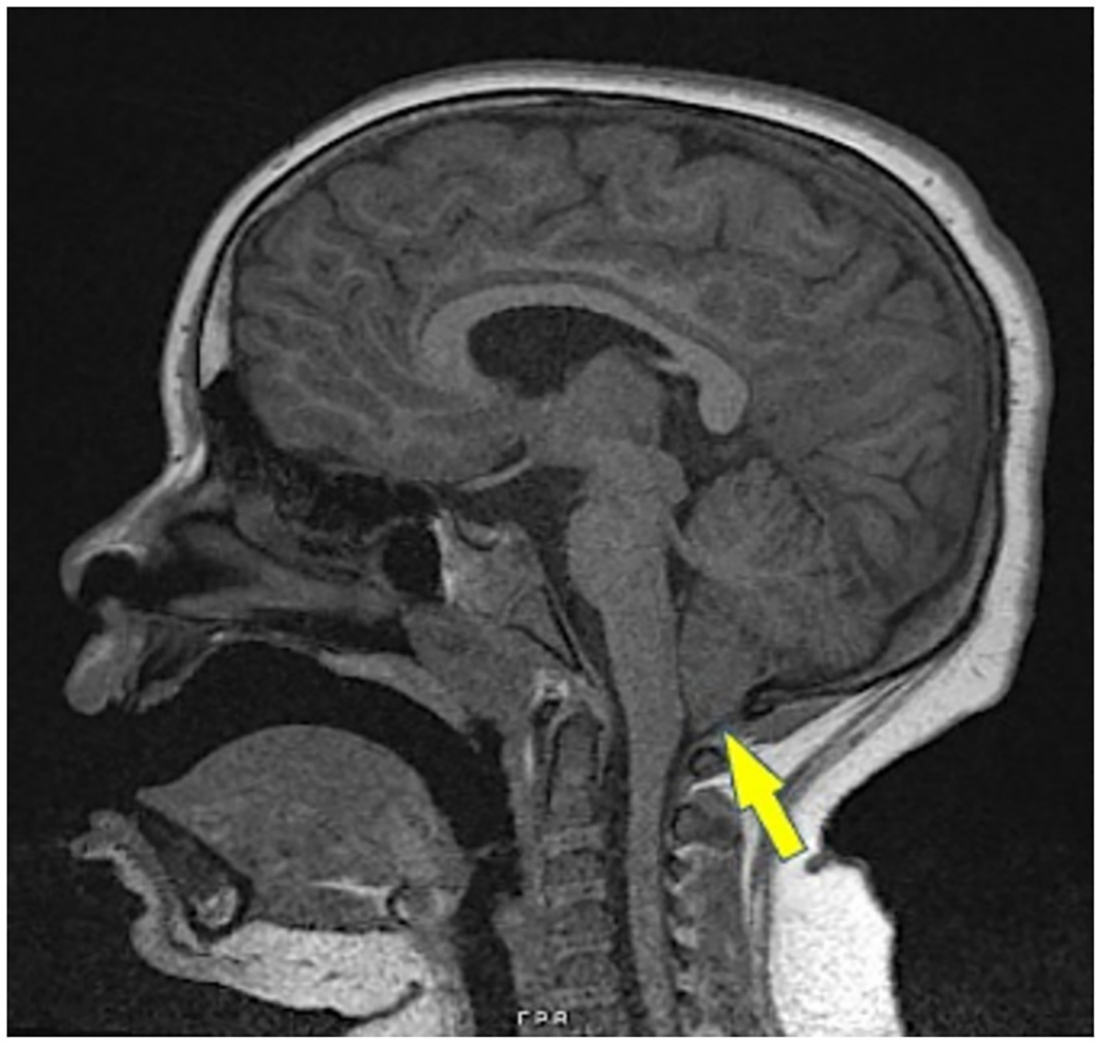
Brain MRI of PHP1A participant. Sagittal MRI image from participant #5. The cerebellar tonsils extend 7 mm below the foramen magnum reflecting Chiari type 1 malformation.
